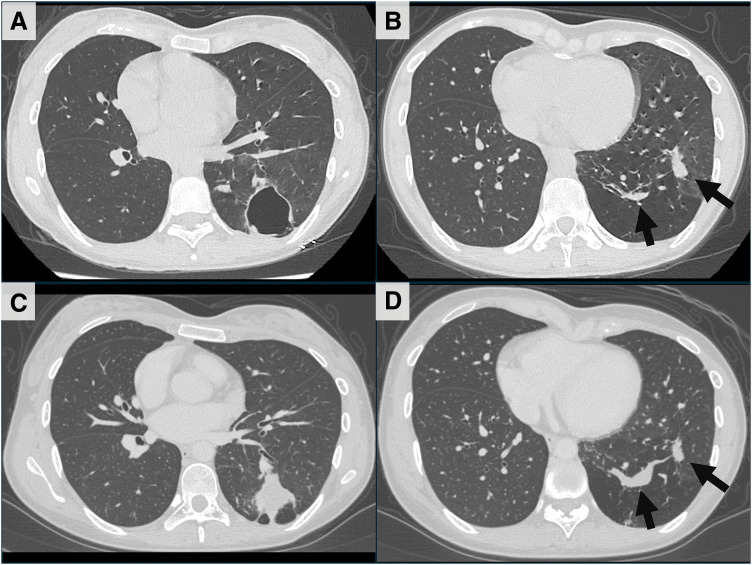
CT images at the time of the initial visit (A, B) and preoperatively (C, D). (A) Lung cyst with a maximum diameter of 40 mm in the left lower lobe. (B) PAVMs (arrows). (C) Lung cyst with fluid accumulation. (D) PAVMs with dilated diameter (arrows).
